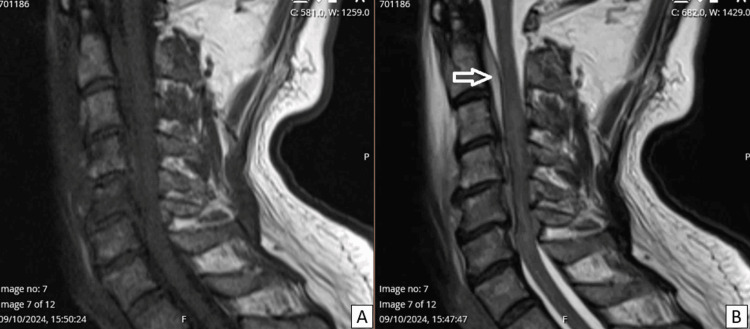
(A) Sagittal T1- and (B) T2-weighted MRI. Spinal epidural abscess is noted anterior do the medulla with high signal intensity (arrow). This inflammation continues to the pre-vertebral compartment.
